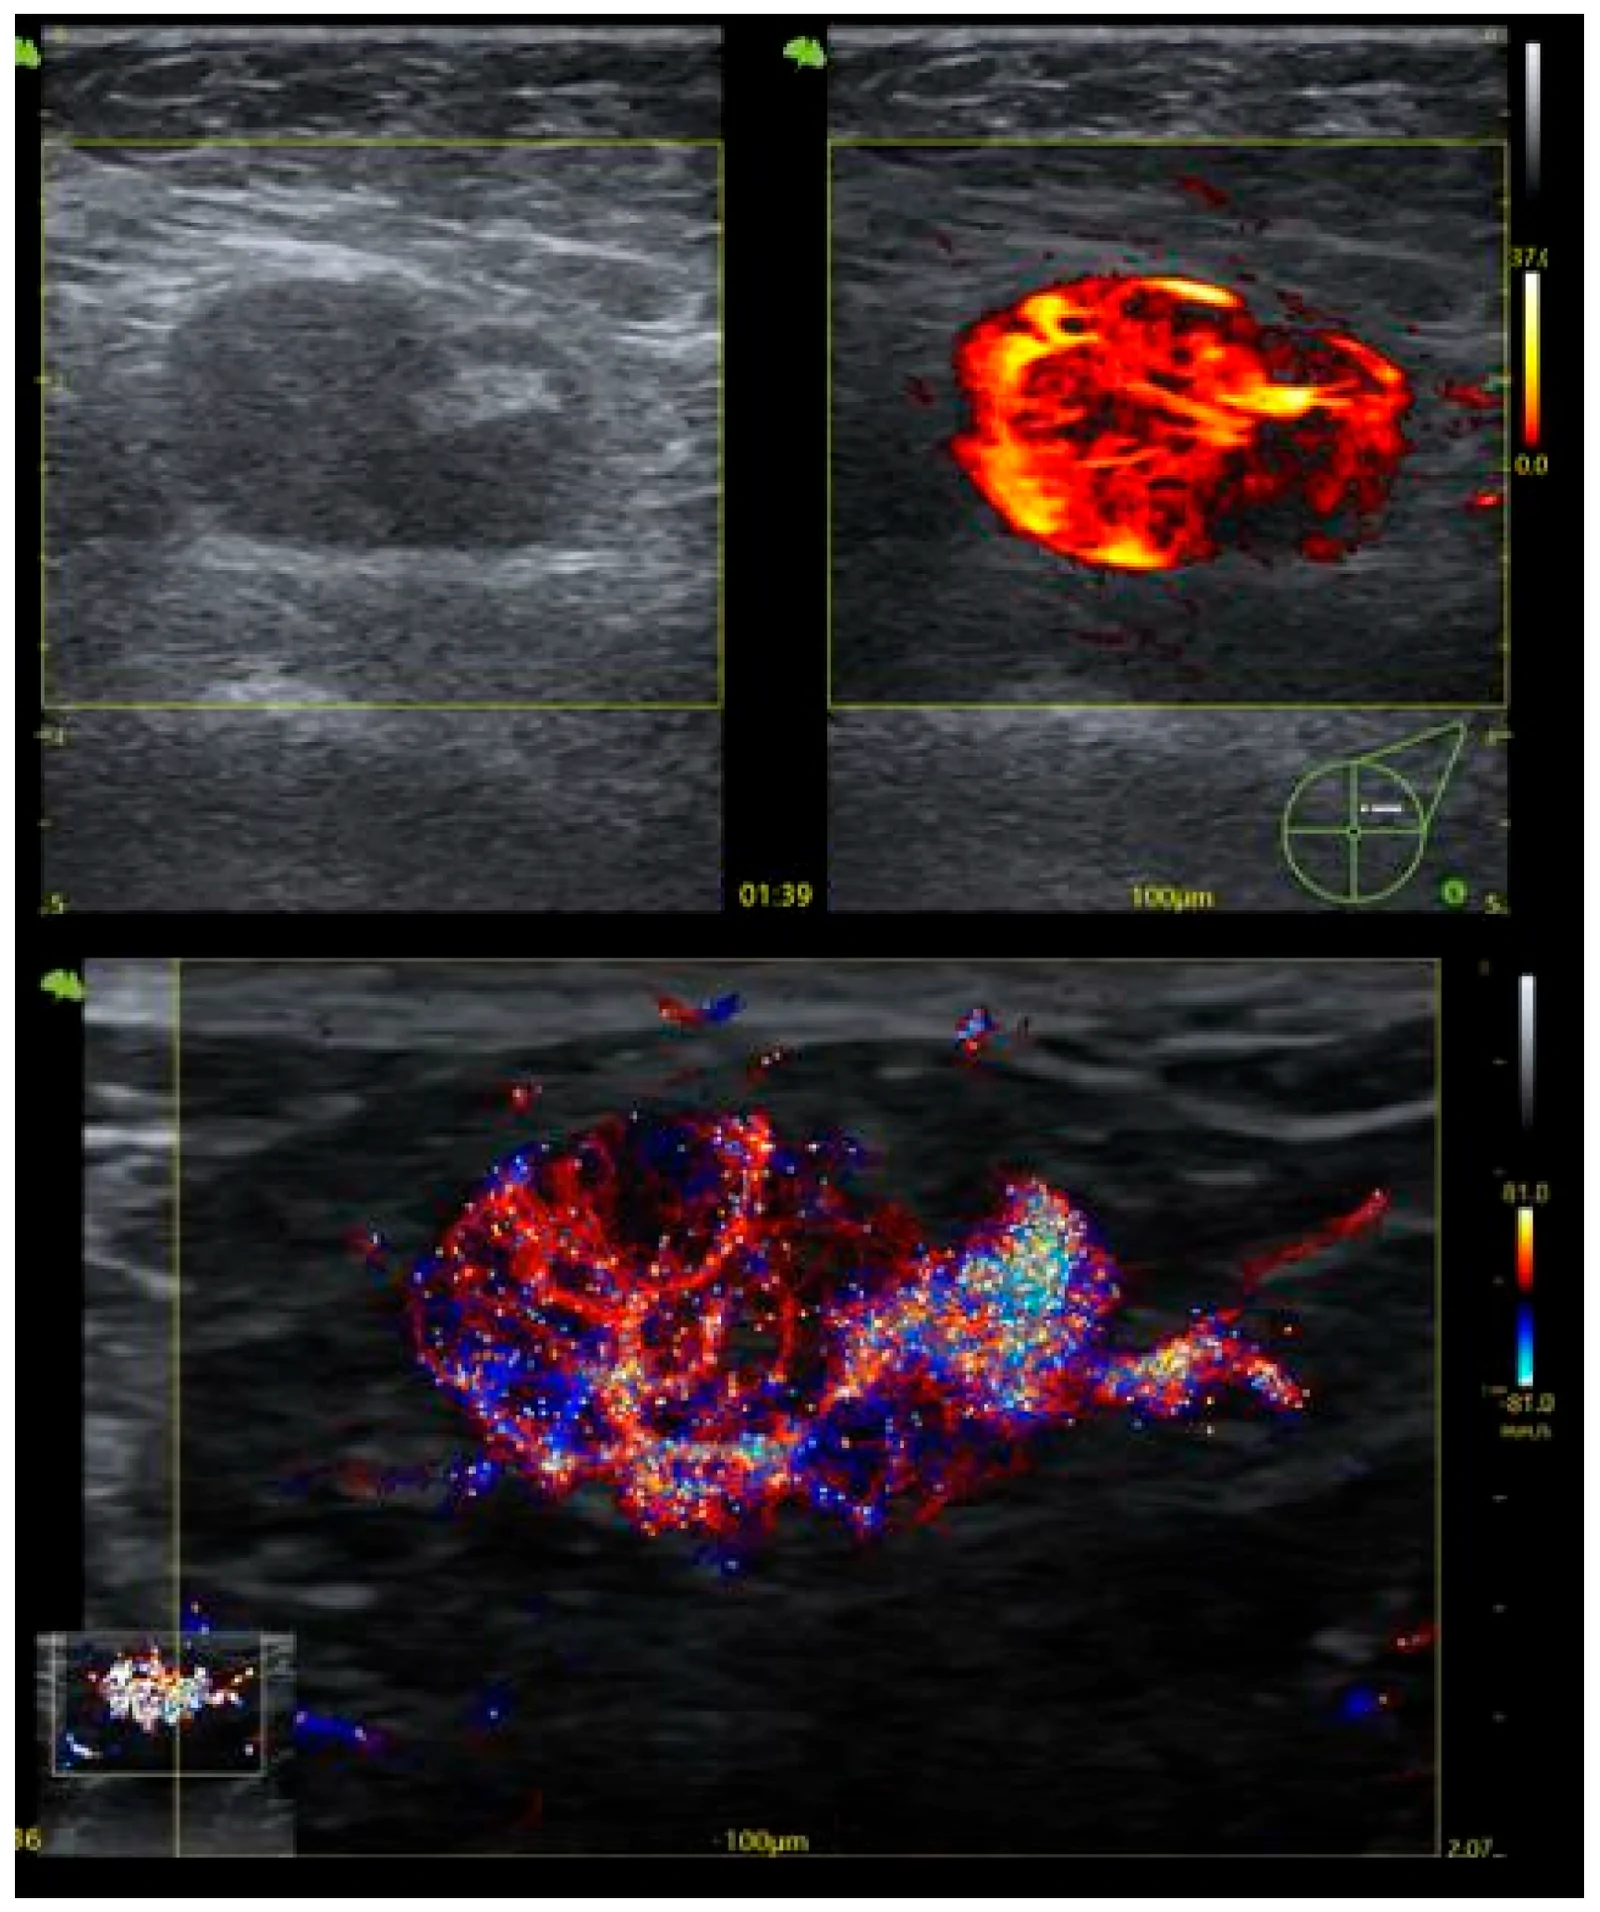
Metastatic axillary lymph node showing grayscale ultrasound and CEUS-URM assessment of hypo- and hypervascular cortical areas.
